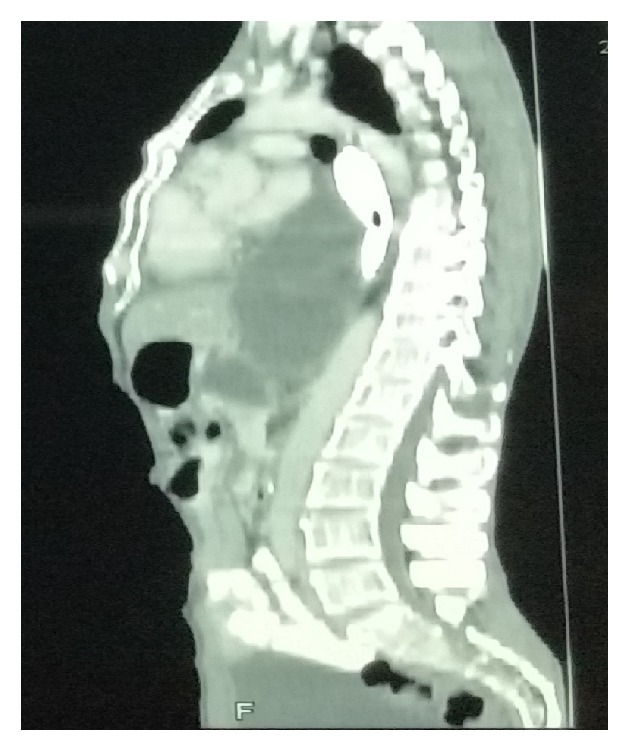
CT chest sagittal view demonstrating the compressive effects on cardiac chambers.
